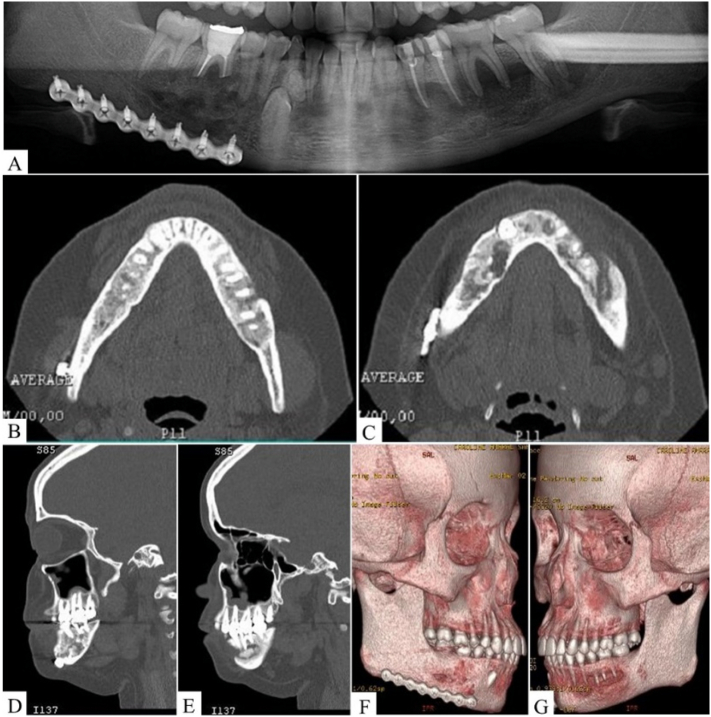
Follow-up imaging analysis. OP performed fifteen days after surgery (A). Axial (B–C) and sagittal (D–E) CT slices after six months showing evidence of bone repair. 3D reconstruction showed bone regeneration of both regions, the right side after two years (F) and left side after six months (G) of follow-up.
